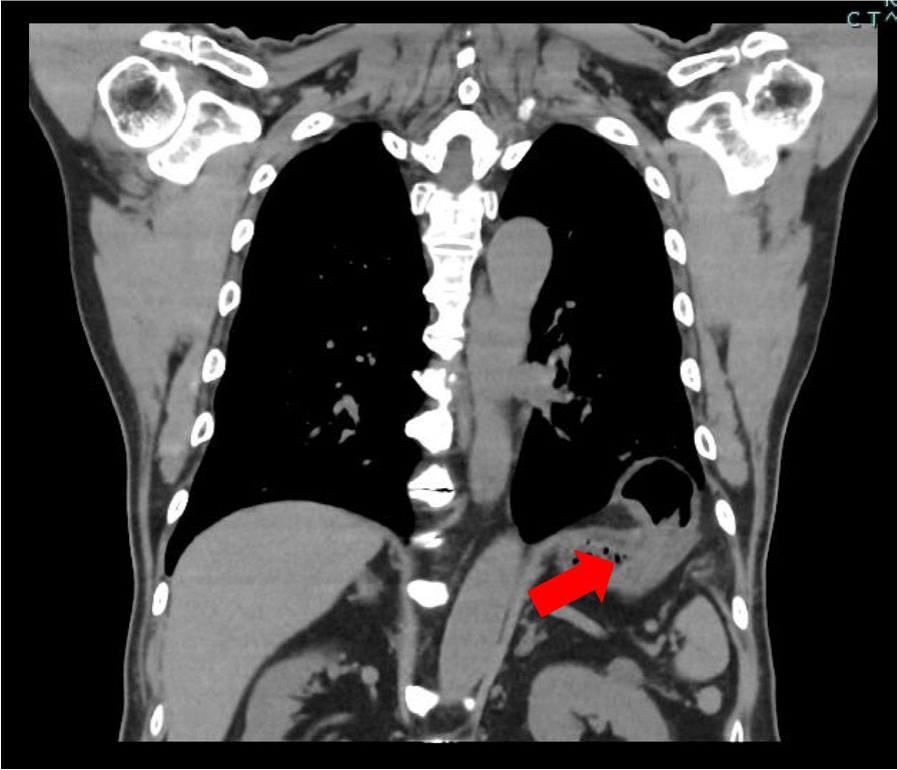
One year after first surgery, we scheduled a routine CT scan. We discovered a left diaphragmatic hernia
